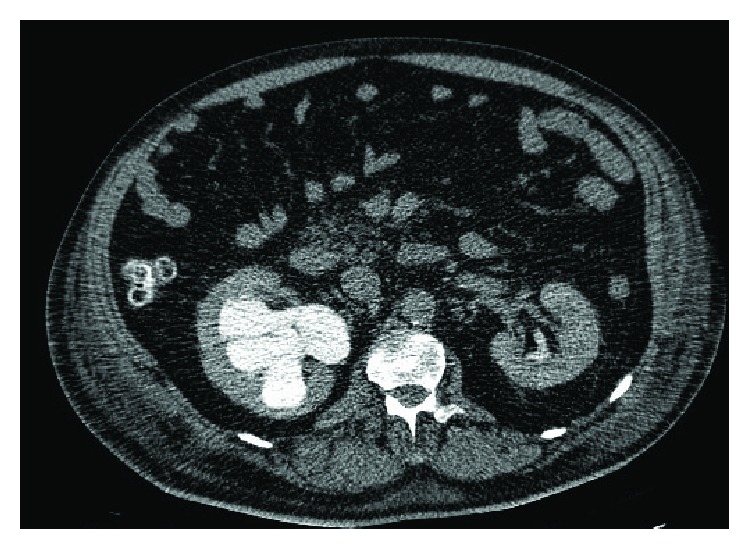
Abdominal-pelvic CT scan with endovenous contrast: right hydronephrosis with ipsilateral renal atrophy.
